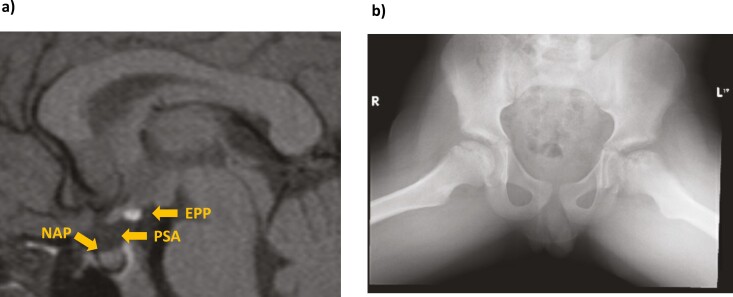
Brain and hip imaging—case 4. (A) Brain magnetic resonance imaging shows EPP, PSA, and NAP. (B) Pelvis and hips radiograph showing (i) marked flattening and sclerosis of the left femoral head in keeping with avascular necrosis, (ii) some sclerosis on the right side, and (iii) posteromedial displacement of the capital femoral epiphysis bilaterally. EPP, ectopic posterior pituitary; NAP, normal anterior pituitary; PSA, pituitary stalk absence.
